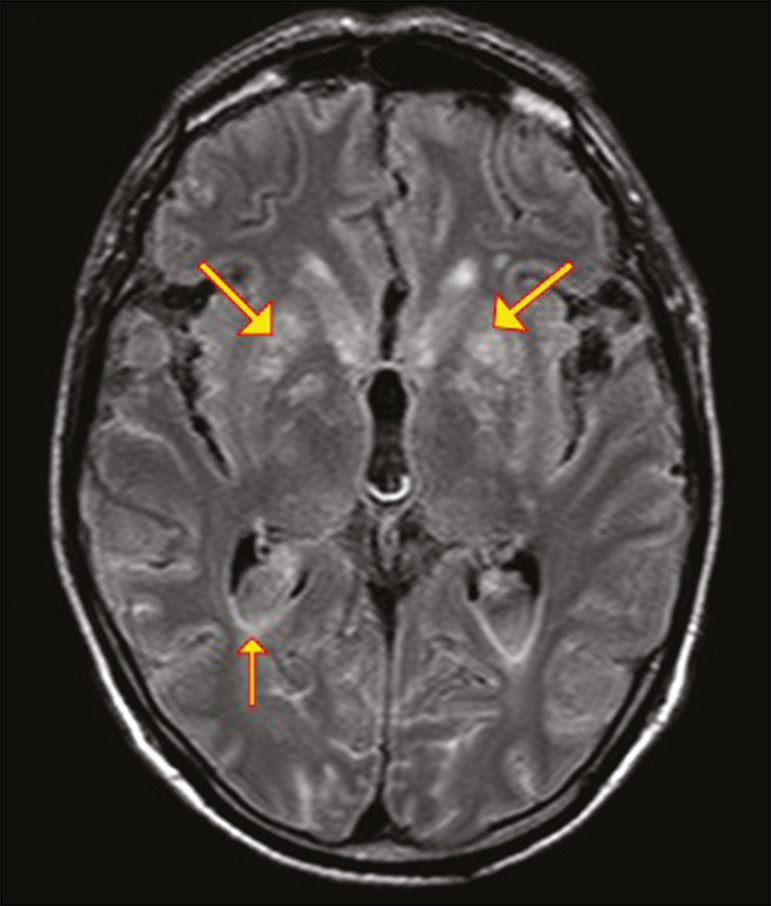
Axial FLAIR MRI sequence showing heterogeneous, predominantly hyperintense lesions in the basal nuclei, consistent with gelatinous pseudocysts (arrows), a classical finding of cryptococcosis of the CNS. Note also the granuloma in the choroid plexus (short arrow) and the hyperintense signal in the leptomeninges.
